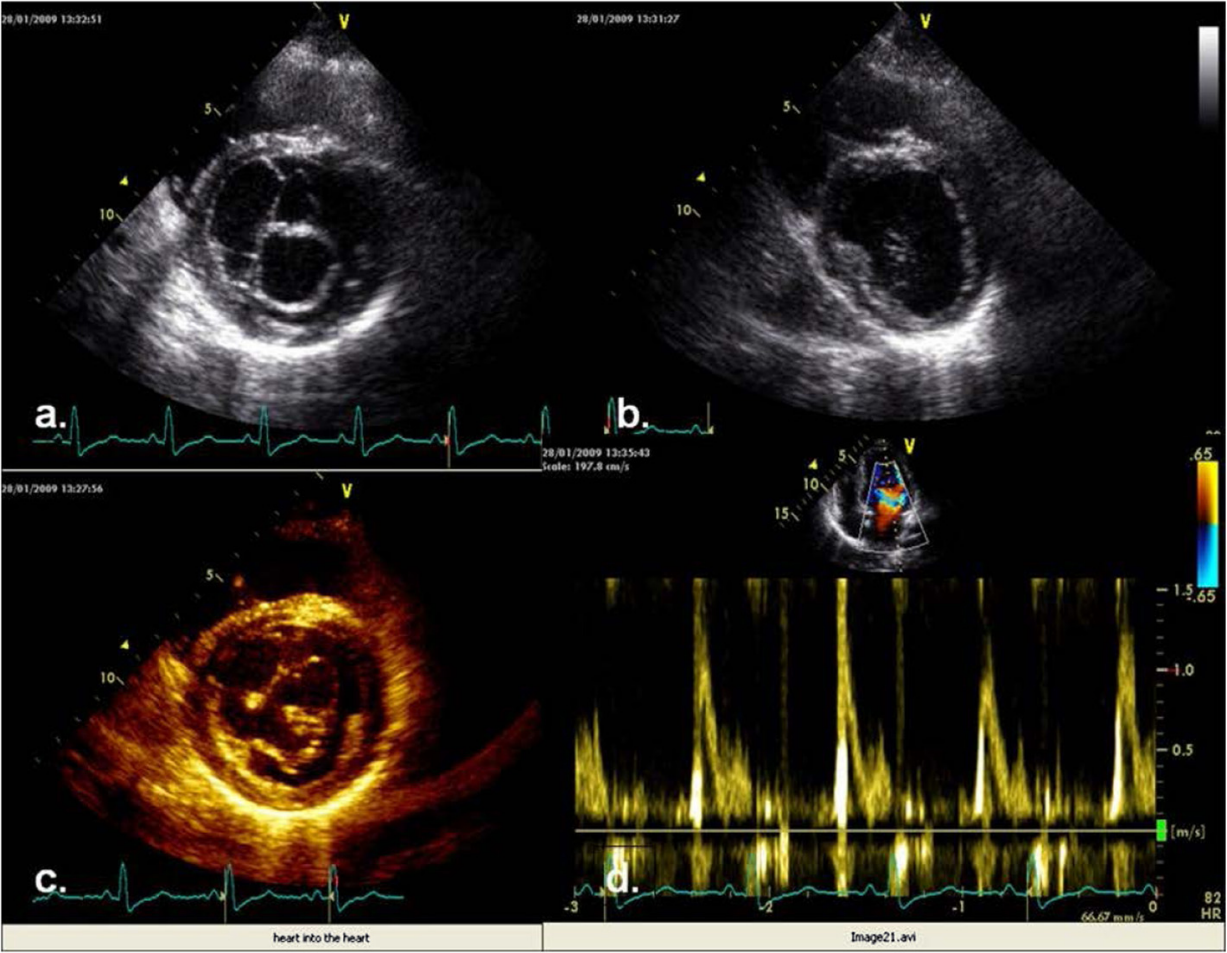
Parasternal long axis view revealed chord meshwork, with normal pulsed-wave Doppler flow. (a) Parasternal long axis view on the level of the mitral valve shows eccentric mitral valve orifice, predominantly oriented to the posteromedial commissure. (b) Parasternal long axis view on the level of papillary muscle shows a single posteromedial muscle. (c) ‘Heart into the heart’ orientation of the chordae. (d) Doppler transmitral flow shows normal relaxation of the left ventricle.
